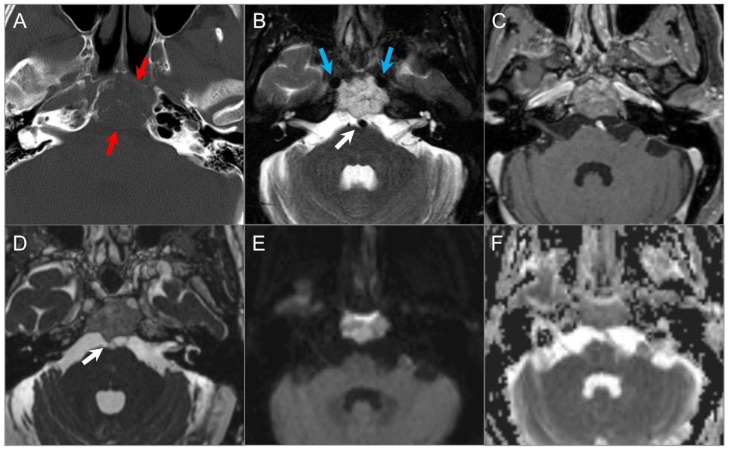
Chordoma. (A) Axial CT image shows a well-defined lytic lesion in the clivus (red arrows) with associated erosion and minimal intratumoral calcifications. (B) Axial fat-suppressed T2W image reveals a marked T2 hyperintensity of the mass with linear hypointense septations, resulting in a ‘soap bubble’ appearance. Note: abutment of the bilateral ICAs (blue arrows). (C) Axial postcontrast T1W image demonstrates heterogeneous enhancement of the lesion. (D) Axial CISS image demonstrates bulging into the prepontine cistern and abutment to basilar artery (white arrow) without definite dural disruption. (E) DWI demonstrates increased signal and (F) ADC map shows similar diffusion characteristics with brain parenchyma in the lesion.
